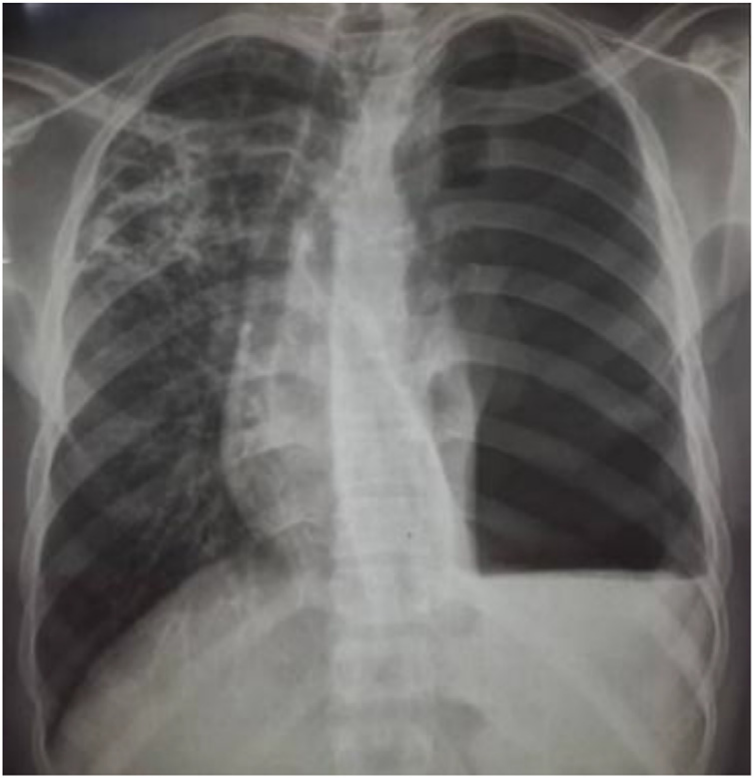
Chest X-ray showing a left hydropneumothorax secondary to extensively drug-resistant tuberculosis in case 1.
